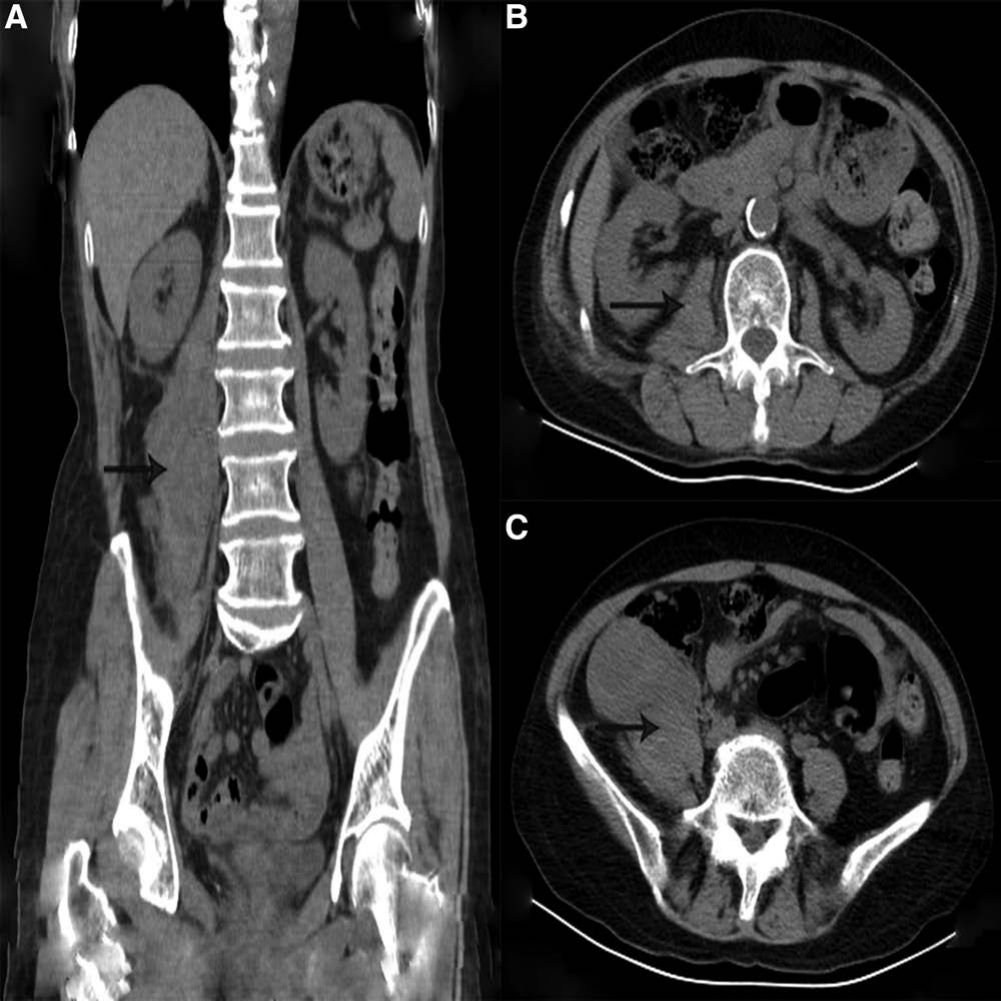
Plain CT image of the abdomen obtained 11 d after the treatment of small needle scalpel. (a) Coronal view; (b) horizontal view at the L2 level; (c) horizontal view at the L5 level.
